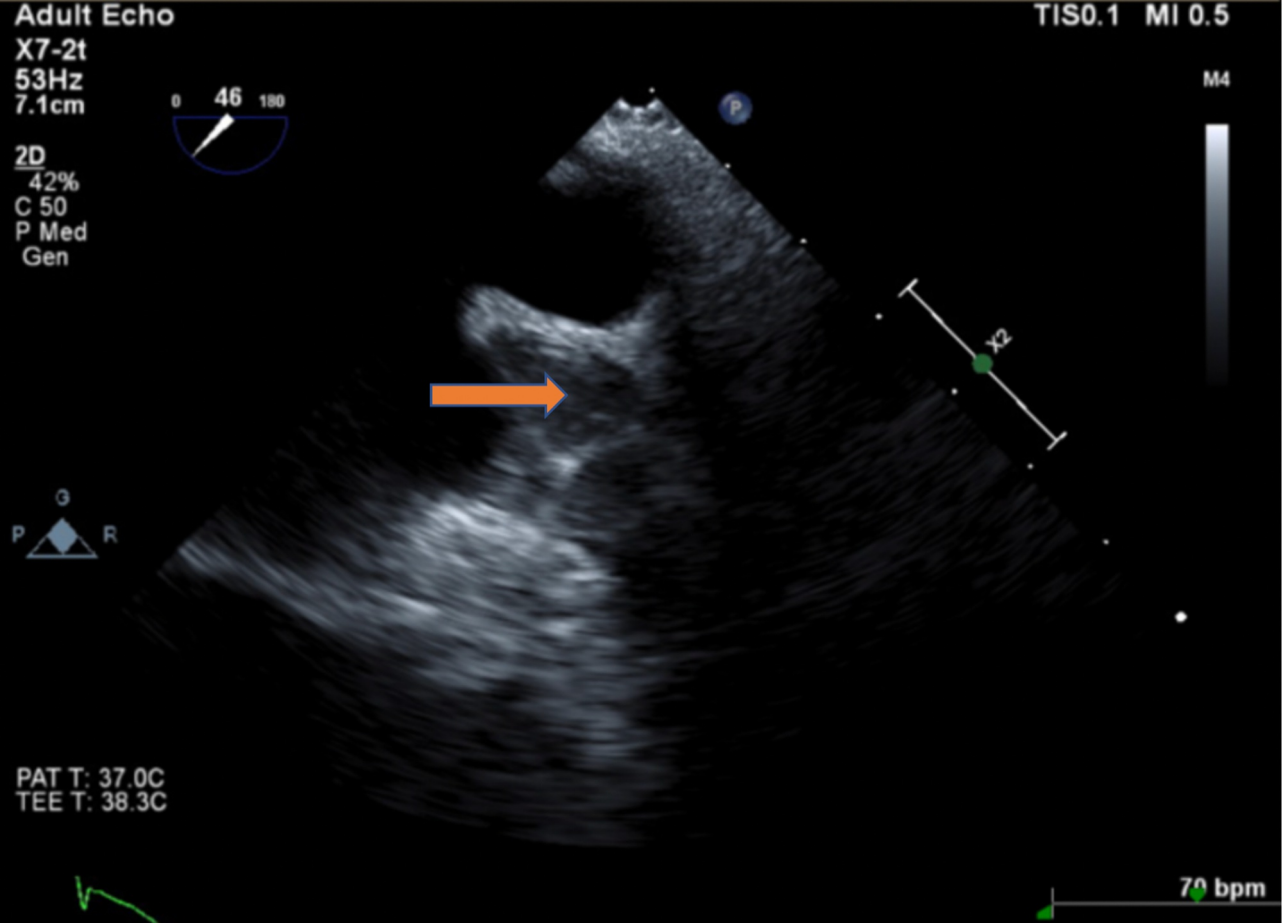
TEE six weeks after Watchman device deployment. Intracardiac thrombus (red arrow) was seen on top of the Watchman device. TEE, transesophageal echocardiogram
